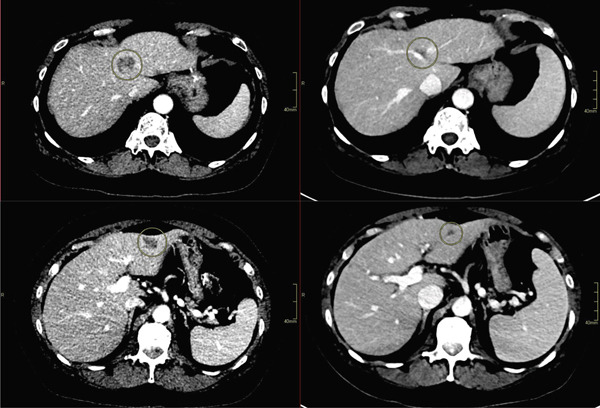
Axial contrast‐enhanced computed tomography (80/150‐kV peak) images demonstrate representative hepatic lesions in Segment IV (top row) and Segment III (bottom row) before (left, pretreatment) and after (right, posttreatment) therapy. Segment IV lesion size decreased from 2.1 × 2.0 × 2.0 cm pretreatment to 1.4 × 1.0 × 0.7 cm posttreatment. Segment III lesion size decreased from 2.0 × 1.2 × 1.3 cm pretreatment to 0.6 × 0.5 × 0.5 cm posttreatment.
